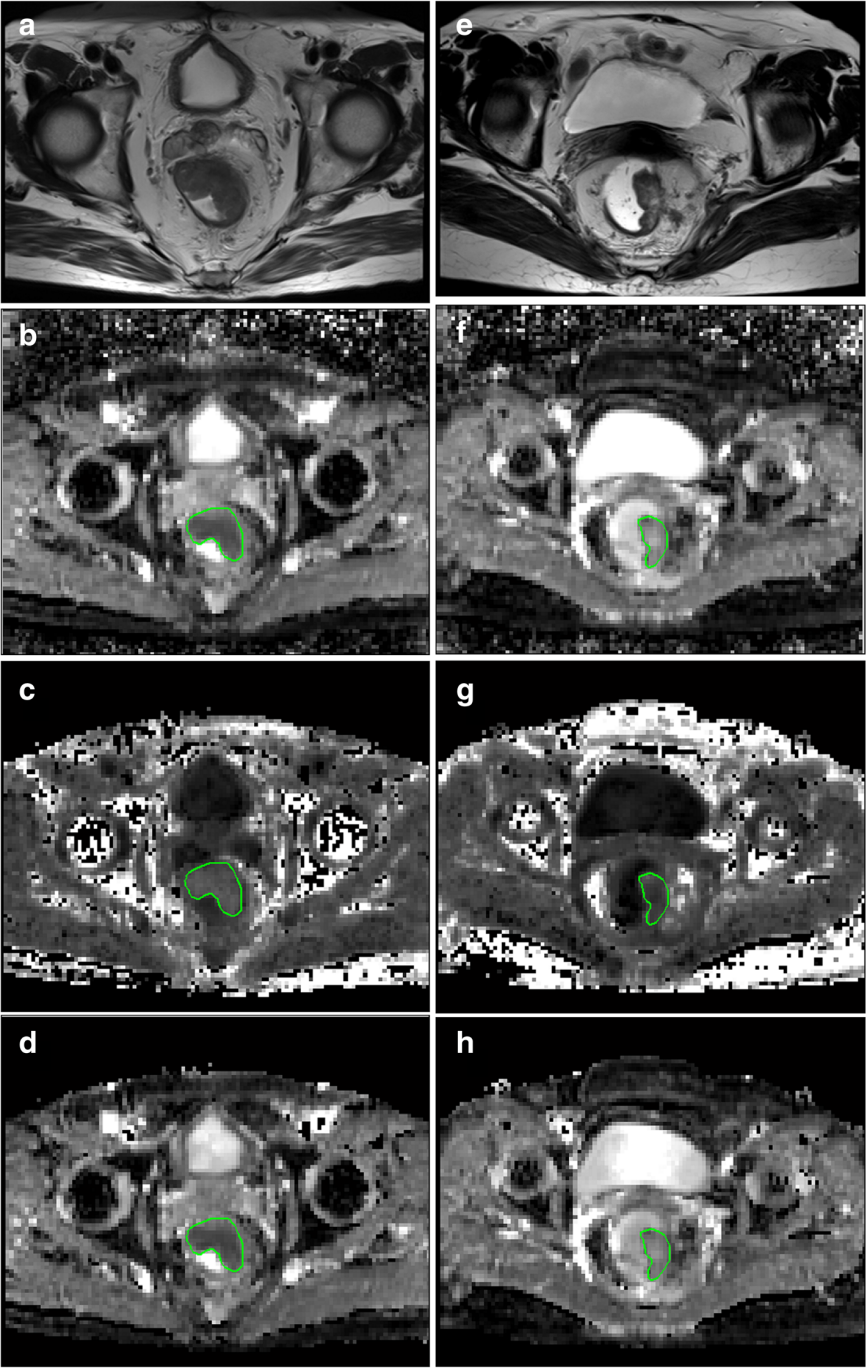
Images above show a 54-year-old man with Grade 2 AC (a-d) and a 72-year-old woman with Grade 3 MC (e-h). Axial T2-weighted MR image shows the AC along the anterior and left wall of the rectum (a). ADC map shows low-signal-intensity tumor (ADC = 1.21 ± 0.92 × 10^− 3^ mm^2^/s, b). MK map shows high-signal-intensity tumor (MK = 1.02 ± 0.01, c). MD map shows low-signal-intensity tumor (MD = 1.19 ± 0.21 × 10^− 3^ mm^2^/s, d). Axial T2-weighted MR image shows the MC along left wall of the rectum (e). ADC map shows high-signal-intensity tumor (ADC = 1.84 ± 0.57 × 10^− 3^ mm^2^/s, f). MK map shows low-signal-intensity tumor (MK = 0.72 ± 0.15, g). MD map shows high-signal-intensity tumor (MD = 1.88 ± 0.31 × 10^− 3^ mm^2^/s, h)
